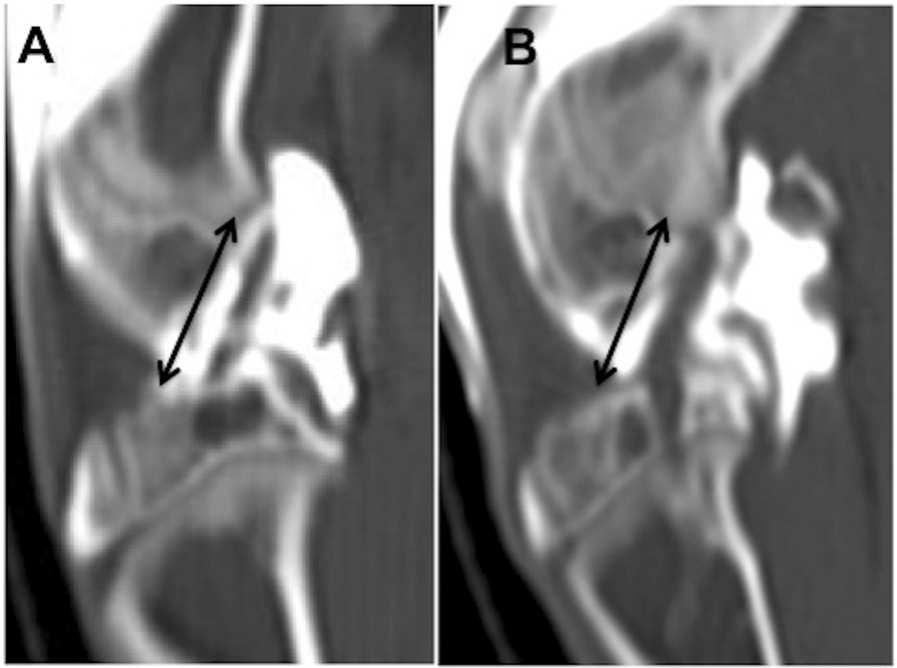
Representative arthro-CT scan for graft placement evaluation. (A) Injection of contrast material into the knee joint revealed the axis of the native ACL. (B) Operated knee exhibiting the same orientation.
